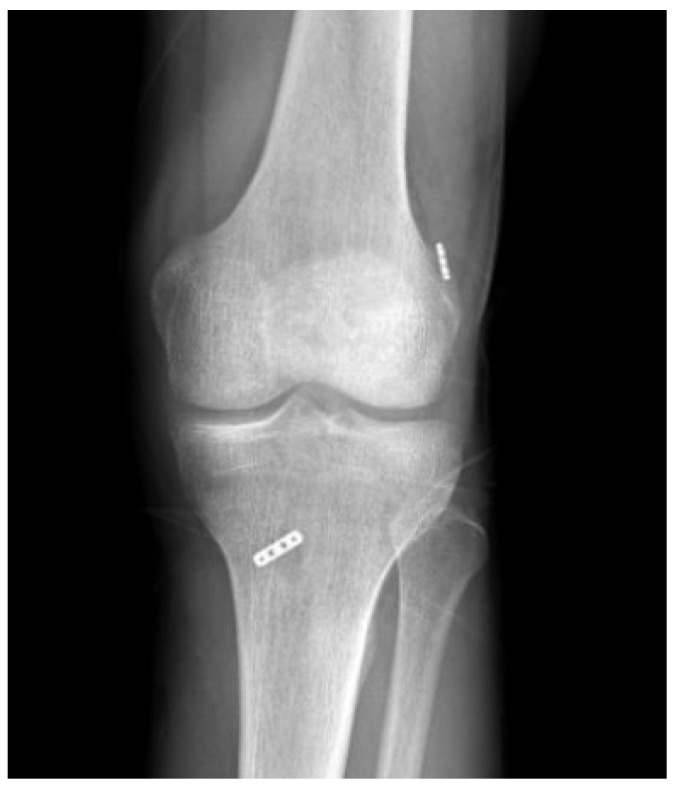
The postoperative radiograph of a patient who underwent anatomical reconstruction using an adjustable suspensory fixation system for ACL reconstruction is presented.
